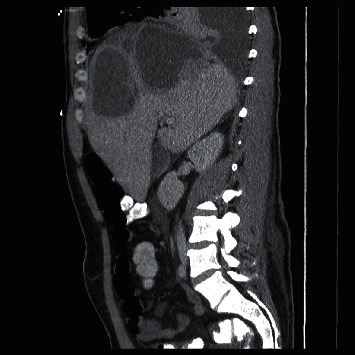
CT chest/abdomen/pelvis with contrast, sagittal view, obtained on hospital day 1 showing large hepatic abscesses.
